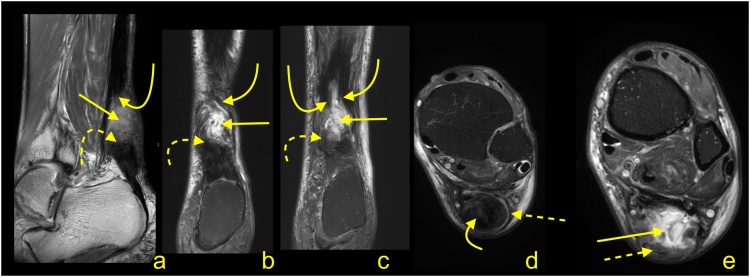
Status after conservative treatment of the Achilles tendon rupture about 4 months ago. MRI revealed that no adhesions are visible between the proximal stump (curved arrow) and distal stump (dashed curved arrow). Very irregular outlines of stumps are noticed. The paratenon is preserved but thick (dashed arrow). A- PD- weighted, b and c- T2- weighted with fat suppression, d and e- PD- weighted with fat suppression.
